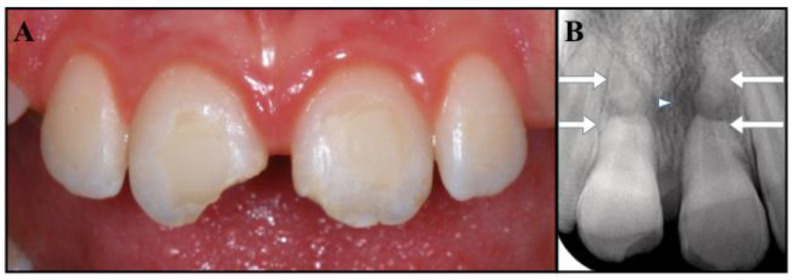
(A) Clinical photograph showing the uncomplicated crown fracture of the incisal third of teeth #1.1 and #2.1. (B) Periapical radiograph showing the apical root fracture of teeth #1.1 and #2.1 (arrowed) and the radiolucency at the fracture line of #2.1 (small arrow).
